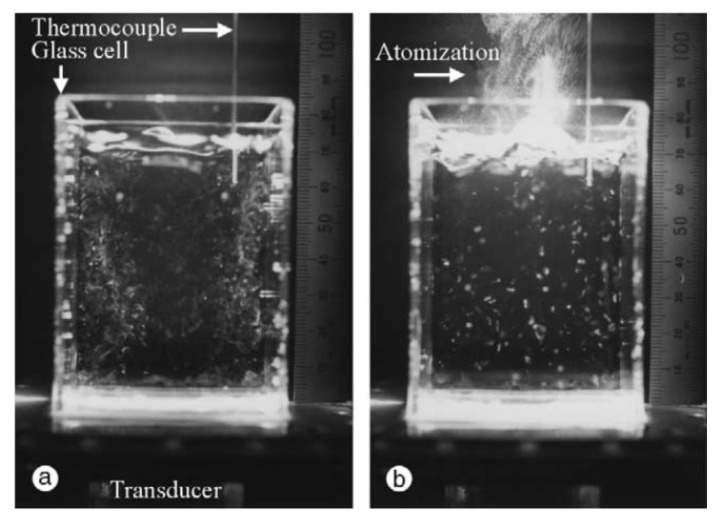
Photographs of cavitation bubbles at 132.2 kHz for the FG outputs of (a) 400 mV_p-p_ and (b) 450 mV_p-p_ taken with a still camera with 33 ms exposure [68]. Reprinted from Ultrasonics, vol. 40, S. Hatanaka, K. Yasui, T. Kozuka, T. Tuziuti, and H. Mitome, Influence of bubble clustering on multibubble sonoluminescence, pp. 655–660, Copyright 2002, with the permission from Elsevier.
